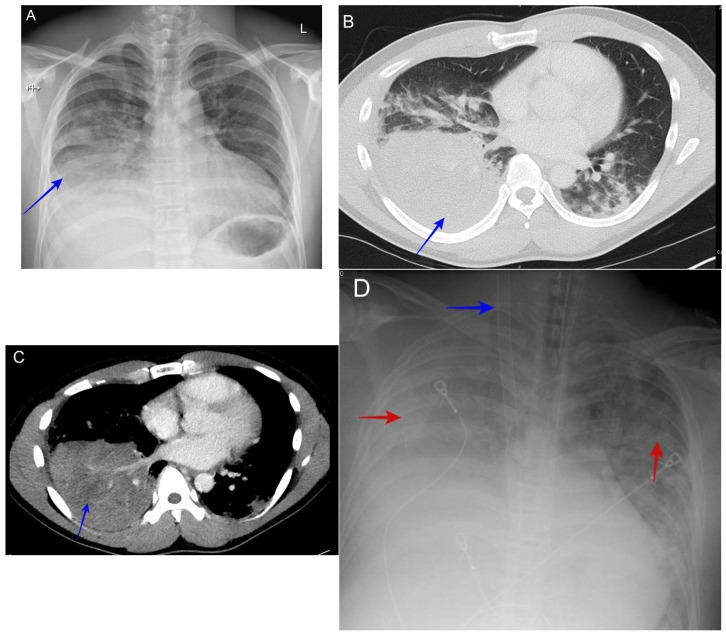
(A) A chest X-ray shows pulmonary coccidioidomycosis mainly involving the right lung (blue arrow). (B) A CT scan shows pulmonary coccidioidomycosis mainly involving the right lung with consolidation (blue arrow). (C) A CT scan shows pulmonary coccidioidomycosis mainly involving the right lung with consolidation and evidence of necrosis (blue arrow). (D) A chest X-ray shows bilateral pulmonary infiltrates (red arrows) coccidioidomycosis with progression to severe ARDS (figure on day 7). The patient was placed on extracorporeal membrane oxygenation (ECMO) therapy (blue arrow shows ECMO cannula). The patient was a 37-year-old Filipino man with no medical history who presented with a fever, cough, and right lower chest pain for 2 weeks. He was initially admitted for CAP therapy, but his cardiorespiratory status deteriorated, necessitating extracorporeal membrane oxygenation (ECMO). Respiratory cultures grew Coccidioides spp.
